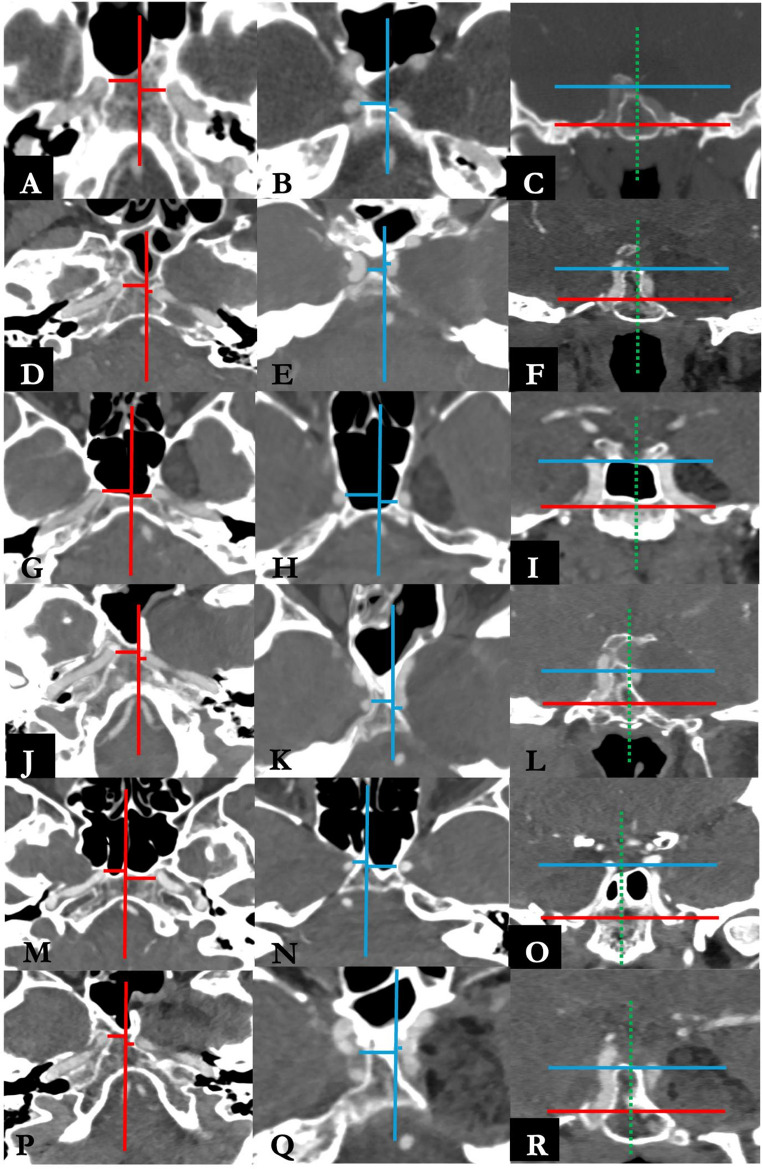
Preoperative angio-CT evaluation of ICA medialization in all the included procedures according to Zoli et al. [28] A, B, C: procedure #1. D, E, F: procedure #2. G, H, I: procedure # 3. J, K, L: procedure #4. M, N, O: procedure #5. P, Q, R: procedure #6. On the axial plane, two slices were selected at the level of C2-C3 genu (A, D, G, J, M, P) and C3-C4 genu (B, E, H, K, N, Q). On the coronal plane (C, F, I, L, O, R), the medialization of the ICA due to the presence of the lesion creates the surgical corridor to access Meckel’s cave.
